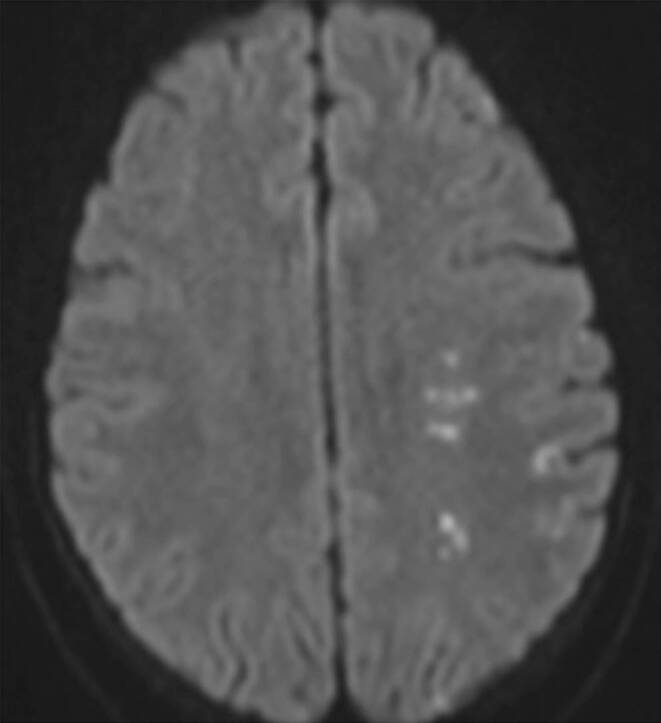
Several small microembolic or possible watershed infarcts were seen on diffusion-weighted imaging. It was unclear if these had occurred prior to the procedure or post-procedure as an MRI scan had not been performed preoperatively. The patient was asymptomatic post-procedure and at last assessment remained at mRS 0
